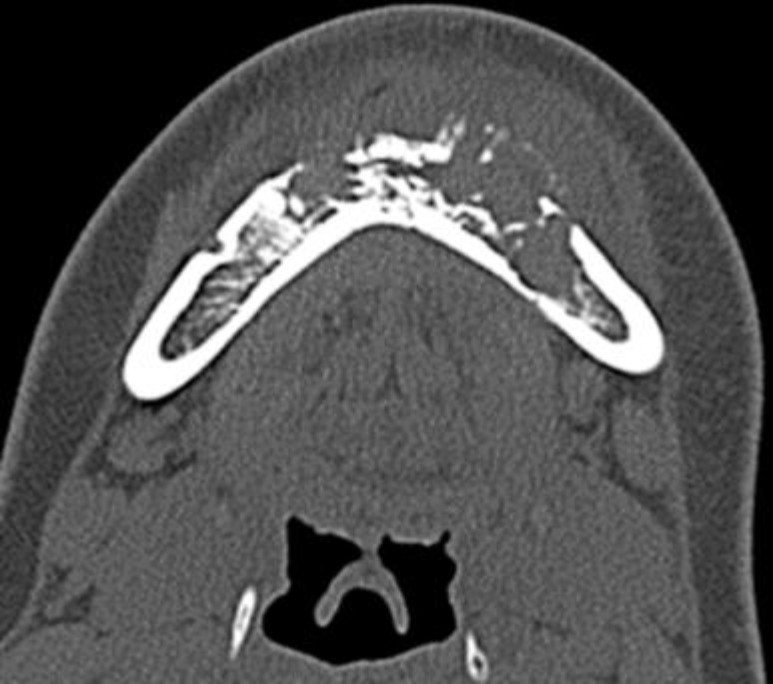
The bone window CT image shows a destruction of mandible
